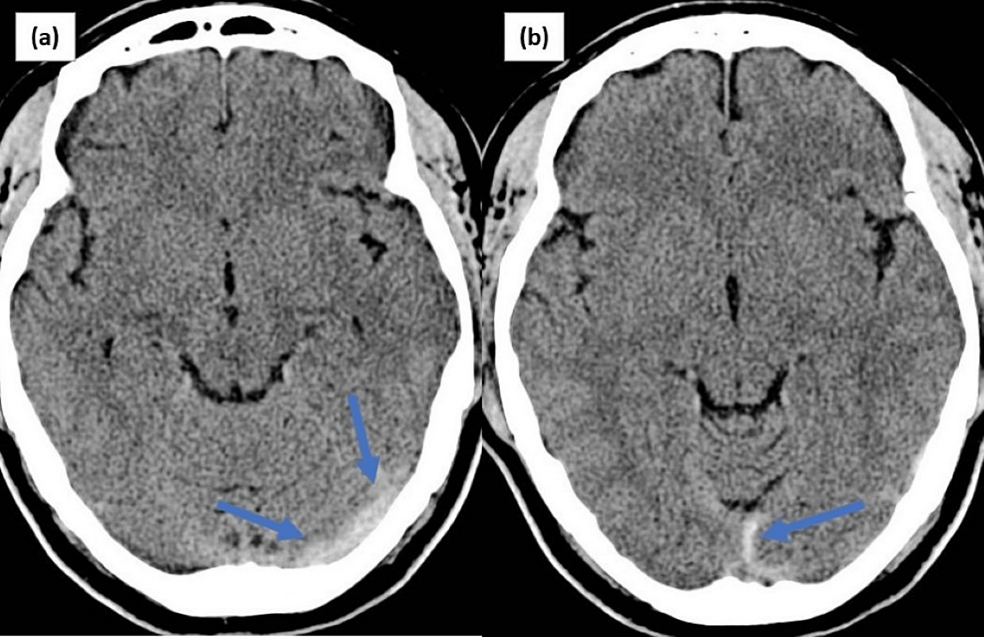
CT brain demonstrating hyperdense area showing thrombosis of left transverse sinus (a) and superior sagittal sinus (b).
